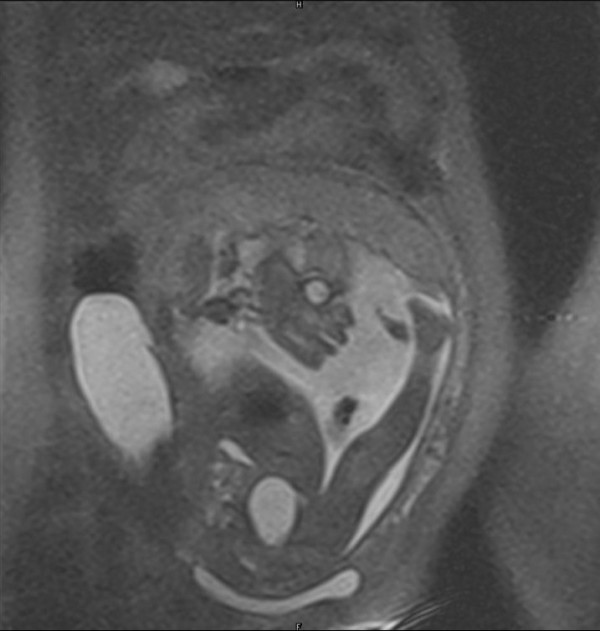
MRI image of fetus with ovarian cyst.
